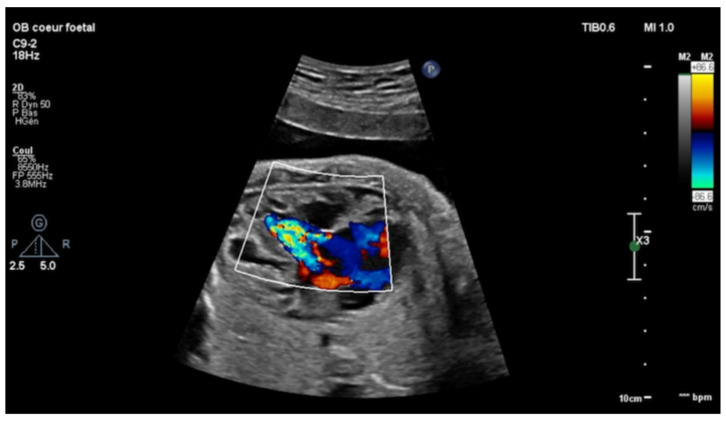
Four-chambers view: color Doppler: tricuspid insufficiency from the apex due to Ebstein’s anomaly.
